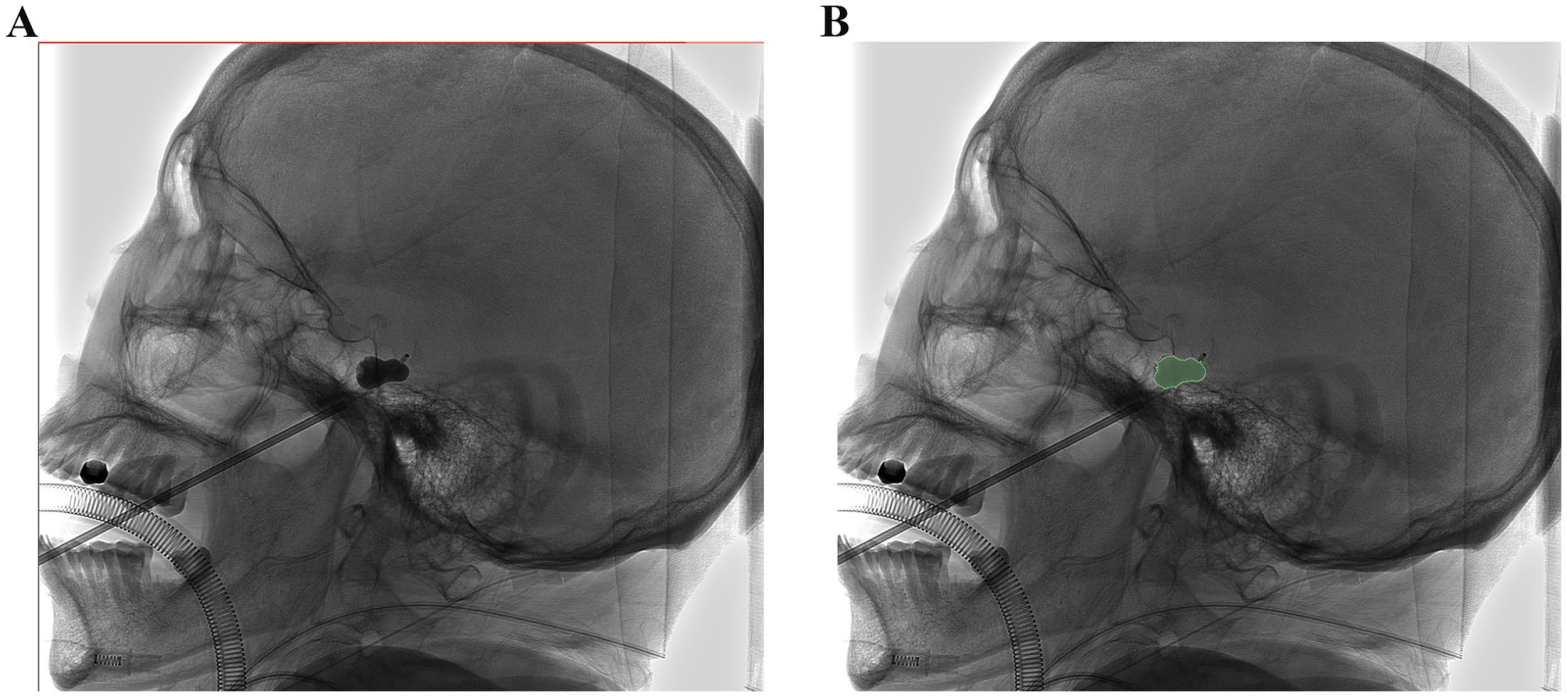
Image morphological feature extraction: (A,B) The ROI was manually drawn on the lateral plain film. Radiomic features were extracted from the radiographs to quantify their shape characteristics.
